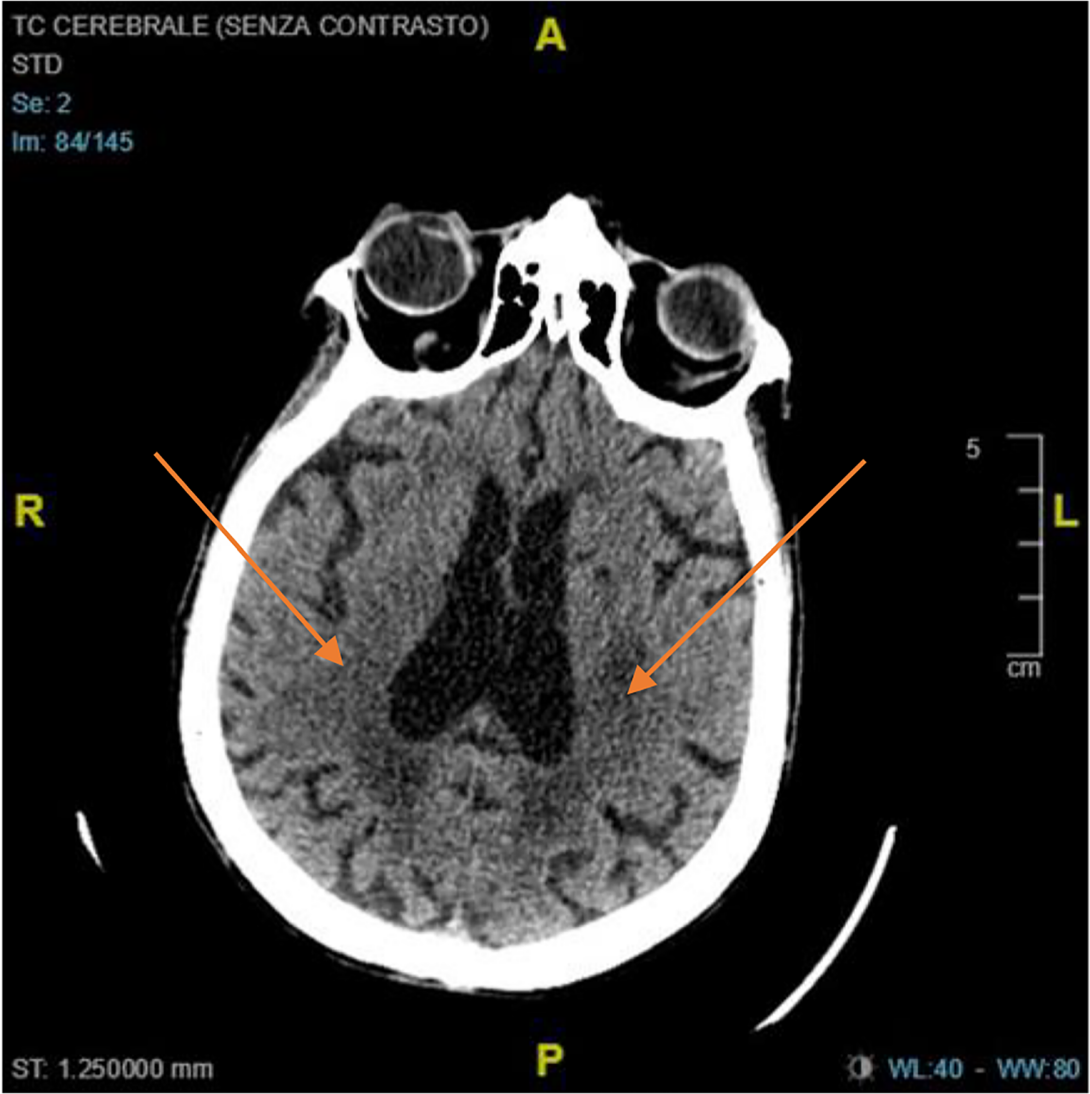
Ten days of brain CT with new periventricular ischemic lesions
